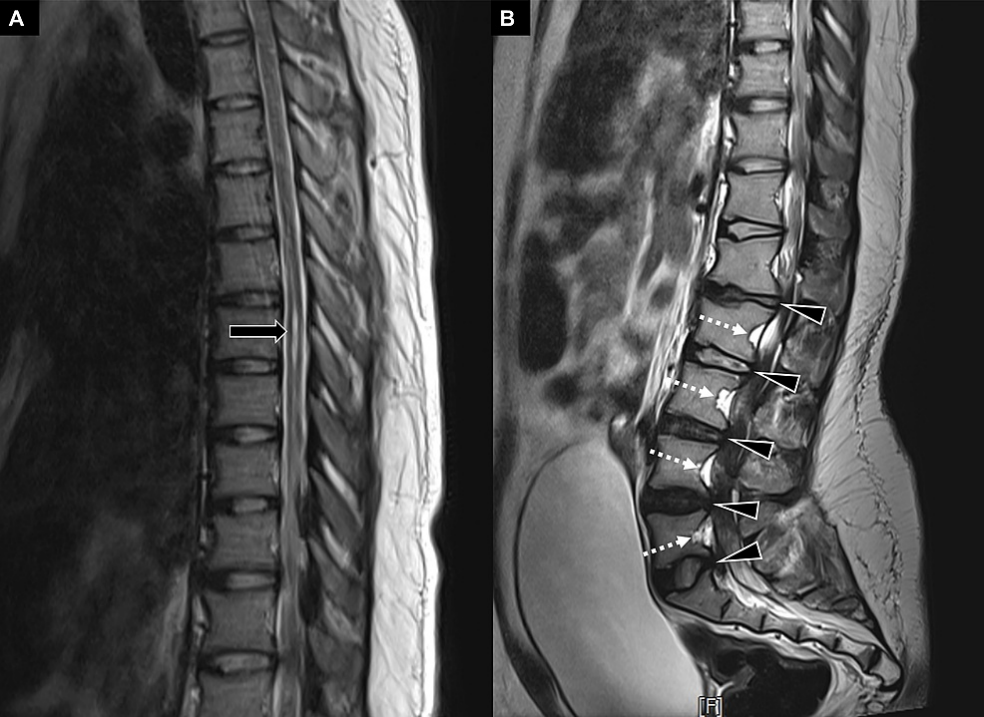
Magnetic resonance imaging for the spine in the sagittal plane Magnetic resonance imaging (T2-weighted sagittal view) shows a syrinx (arrow) at the level of the 6th - 8th thoracic vertebrae (A). Also note the excessive lumbar lordosis, posterior scalloping of vertebral bodies (dashed arrows), protruding intervertebral discs (arrowheads), and lumbar spinal stenosis (B).
